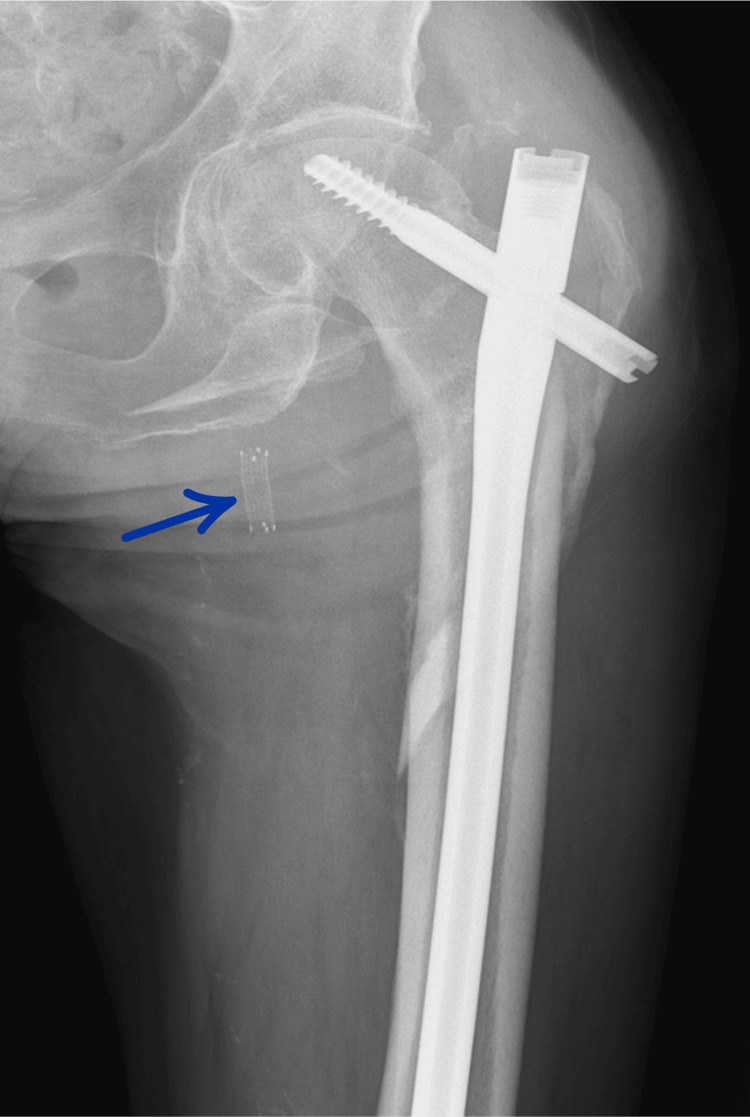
Three-week postoperative radiograph showing the vascular stent in place and early signs of fracture consolidation
